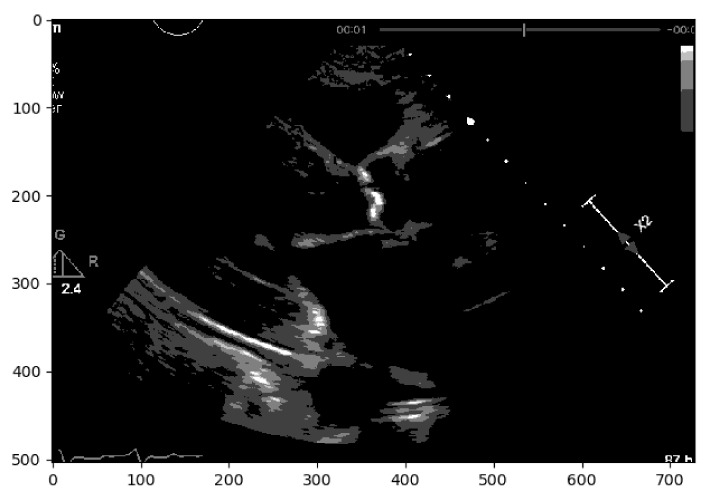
Echocardiography image with region-based segmentation.
